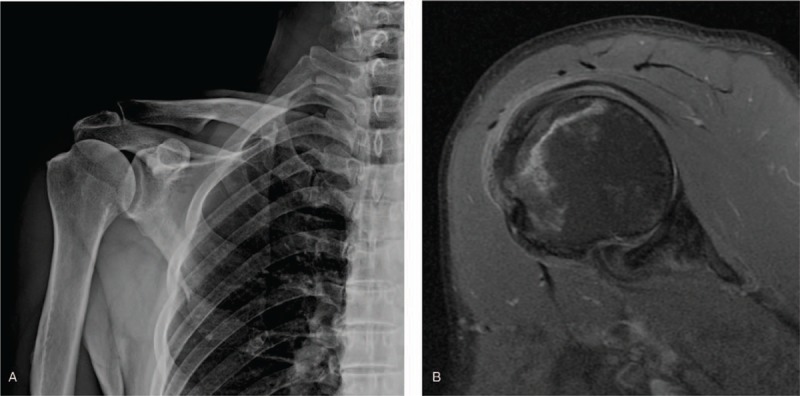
(A): A focal wedge-shaped defect on the superior lateral aspect of the right humeral head. (B): Right shoulder magnetic resonance imaging of the biceps axial view. The proton density fat-suppressed image shows a double-line sign with edema on the greater tuberosity region, a lesion compatible with osteonecrosis.
